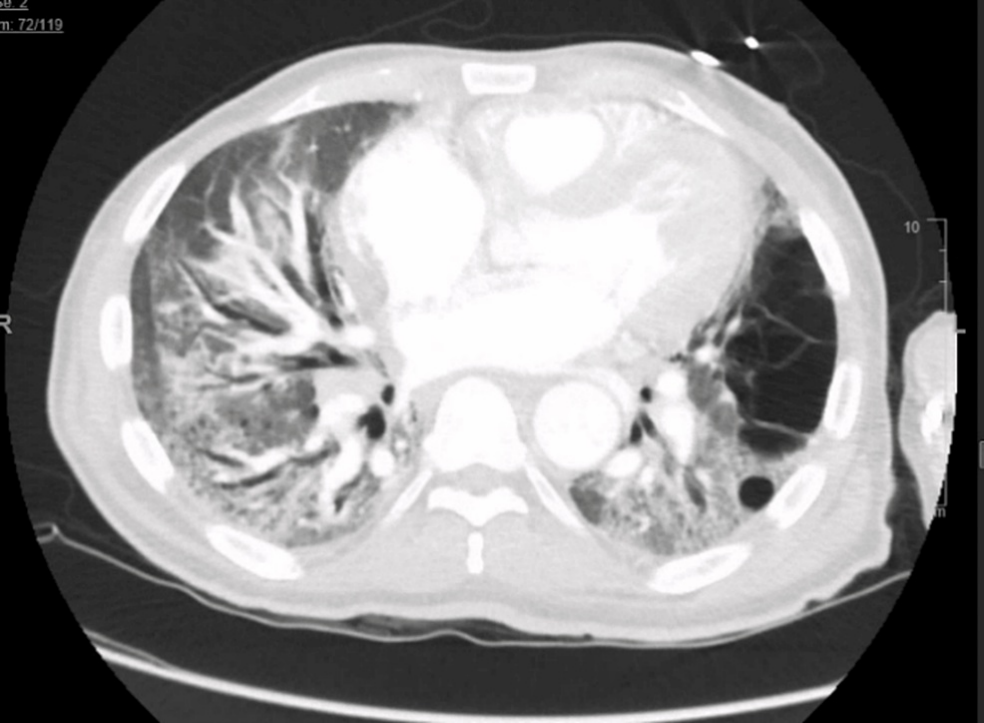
CT chest 1 year after chemotherapy showing advanced changes of honeycombing and traction bronchiectasis suggesting grade 4 pulmonary fibrosis
